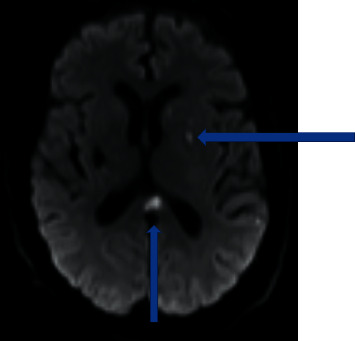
MRI diffusion weighted imaging with acute infarcts in the splenium of the corpus callosum and the posterior superior frontal lobe.
